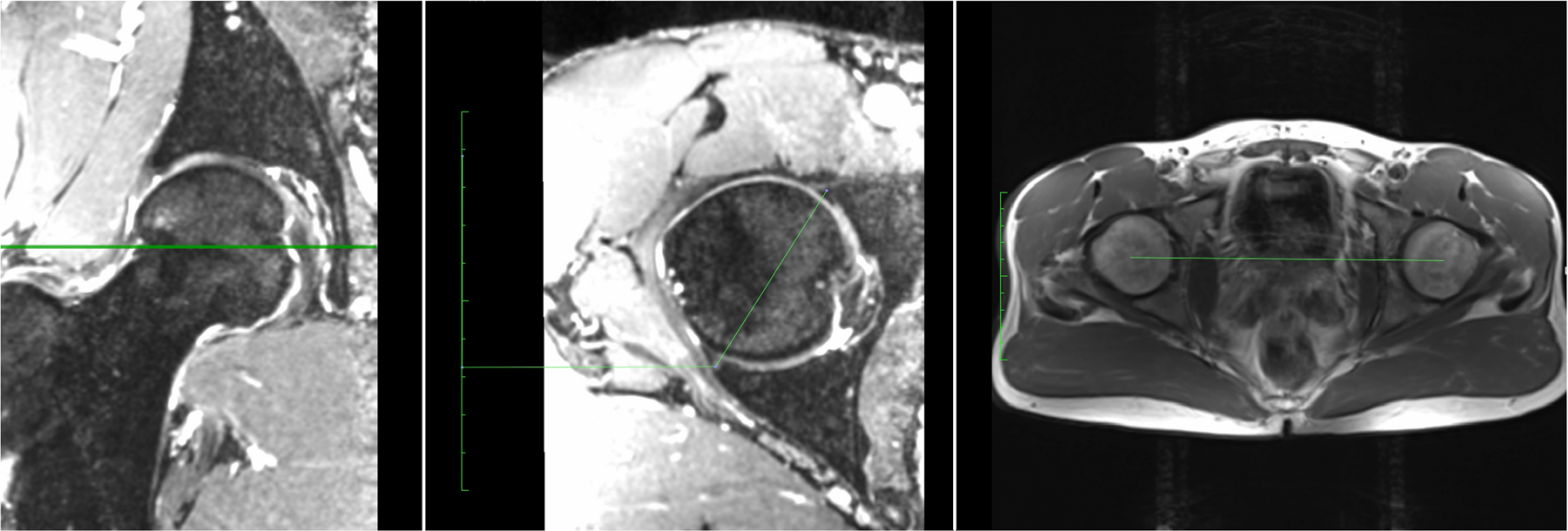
Acetabular version measurement. The acetabular version was measured using axial hip MRI sequences at the level of the center of the femoral head, with the line connecting the center of both femoral heads used as a reference point. MRI, magnetic resonance imaging.
